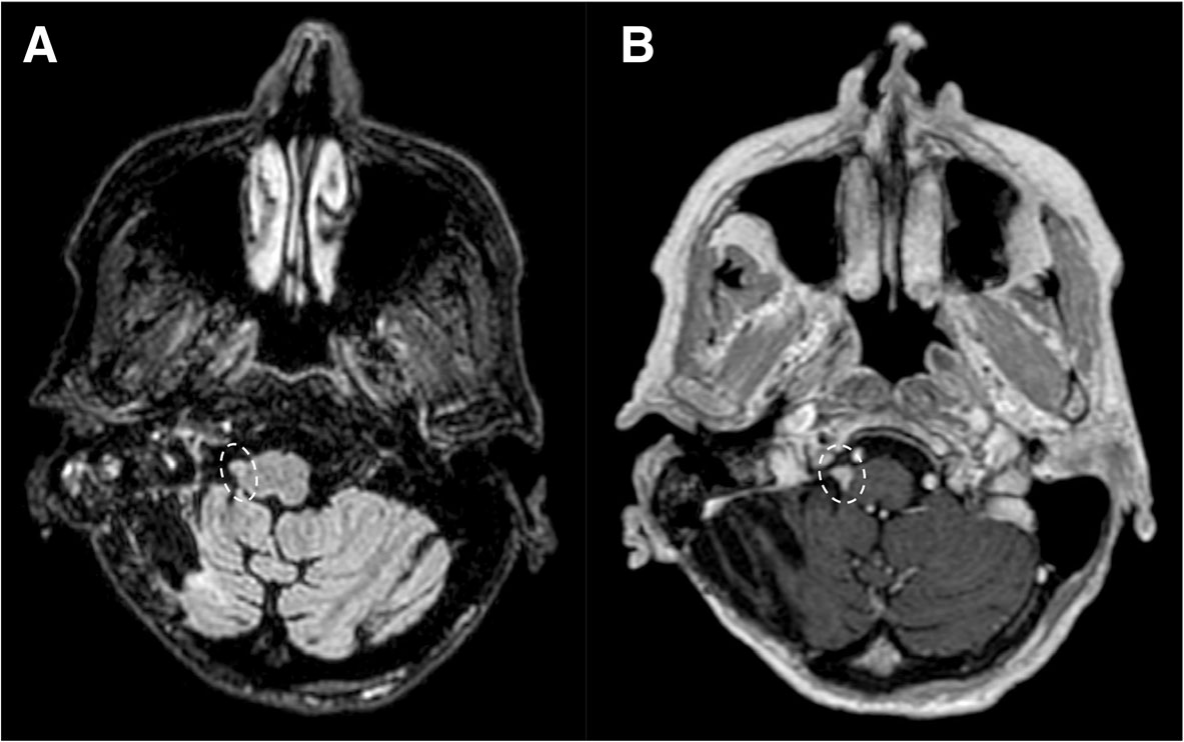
Small glossopharyngeal neurofibroma. Patient with NF1. MRI FLAIR (a) and T1 post-gadolinium (b) sequences, axial plane, demonstrate a mildly hyperintense small lesion (dotted circles) with avid enhancement located on the right post-olivary sulcus
